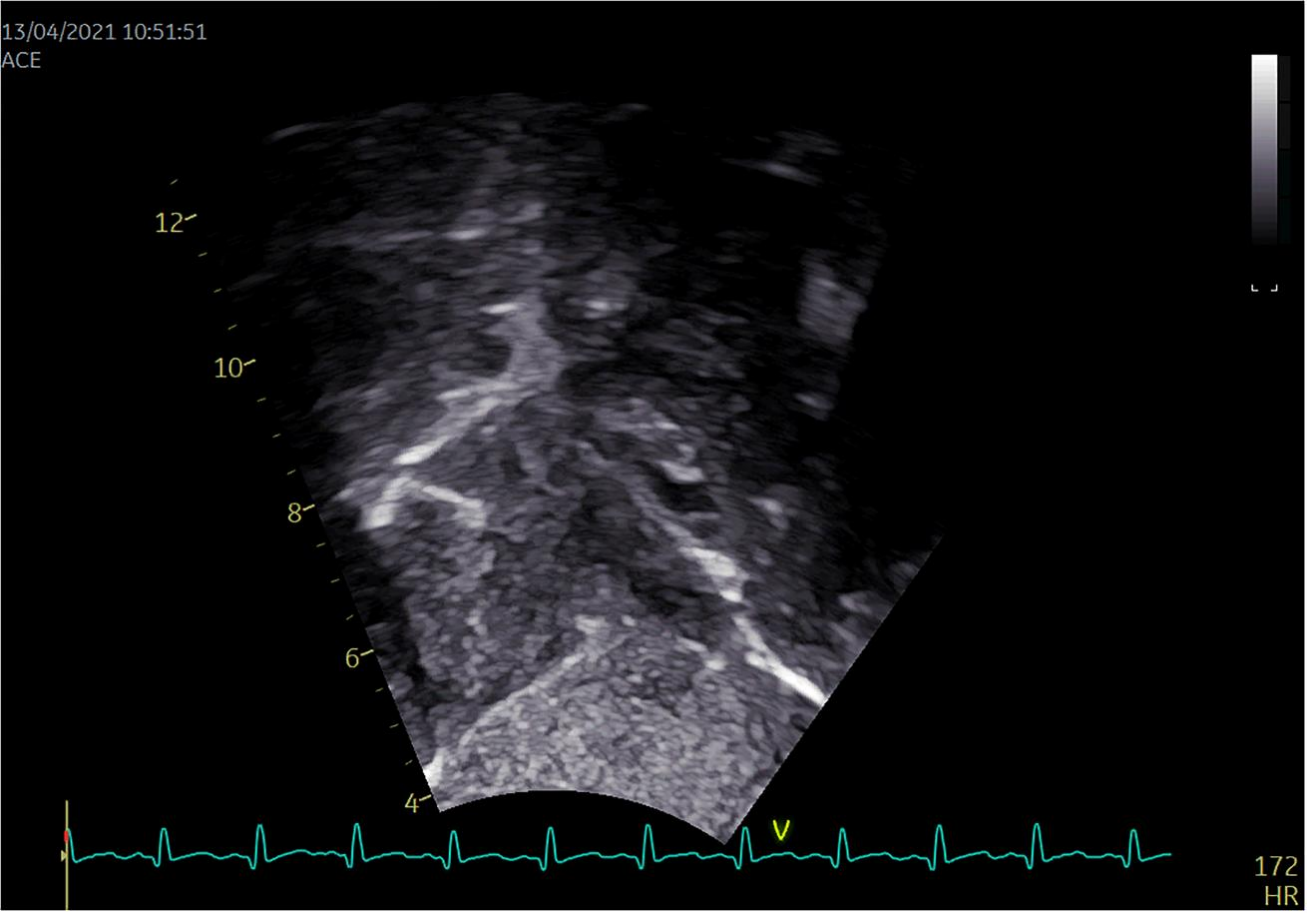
Pretreatment contrast transthoracic echocardiography image.
